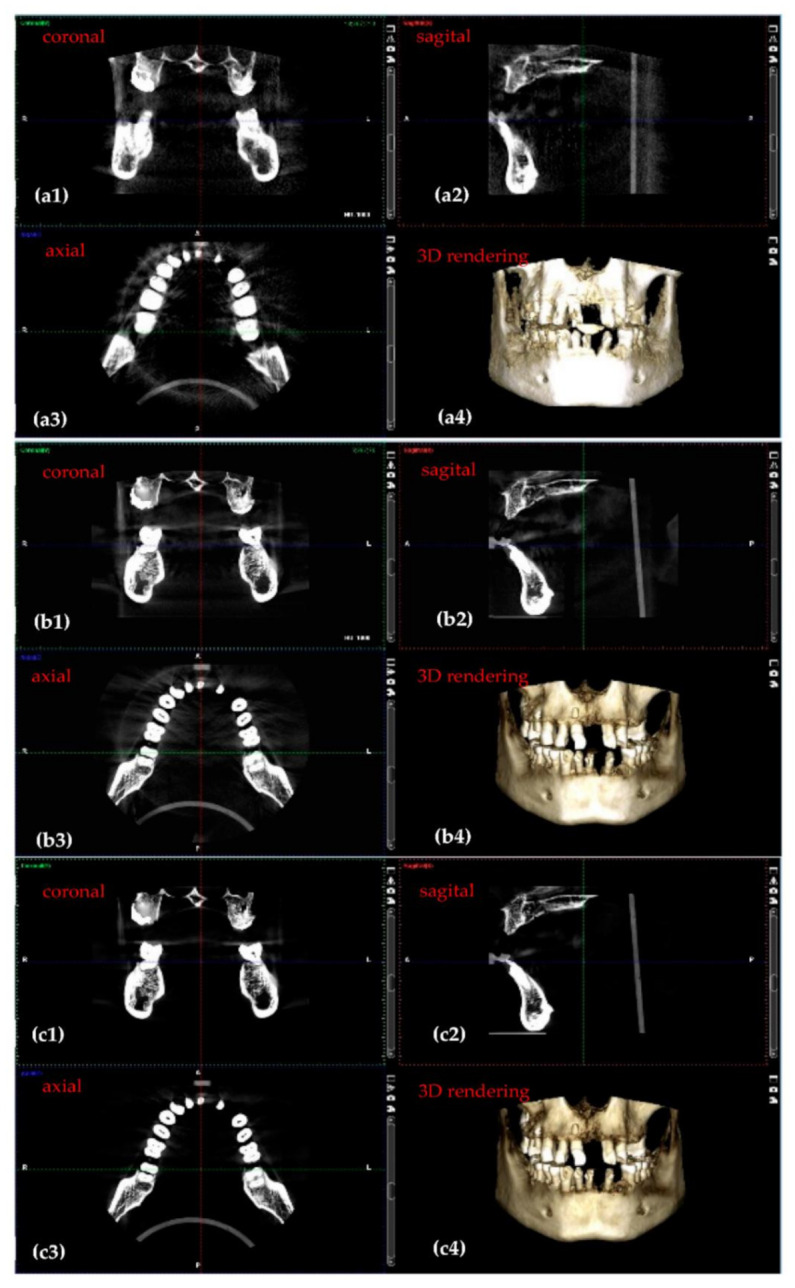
3D CBCT images obtained through the optimization process. The steps of this process include: (a) first 3D CBCT images with the smallest voltage and current values (b) an intermediary 3D CBCT; (c) the most high-quality 3D CBCT obtained. The four corresponding images in each panel represent: (1) coronal view; (2) sagittal view; (3) axial view; (4) 3D rendering.
